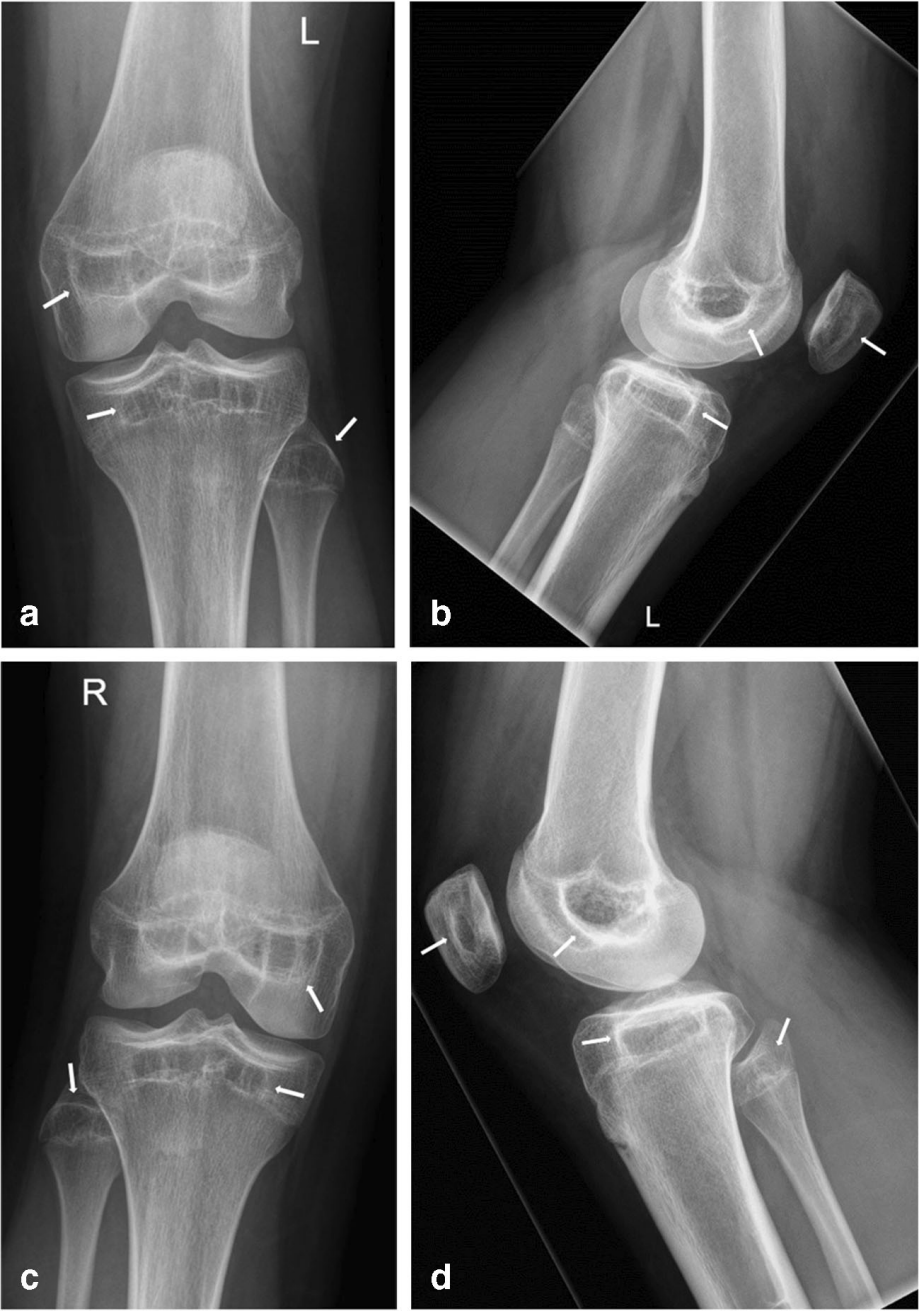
a–d PA weight-bearing and lateral conventional radiographs of both knees. Arrows indicate epiphyseal/acrophyseal growth arrest lines, outlining the former SOC
